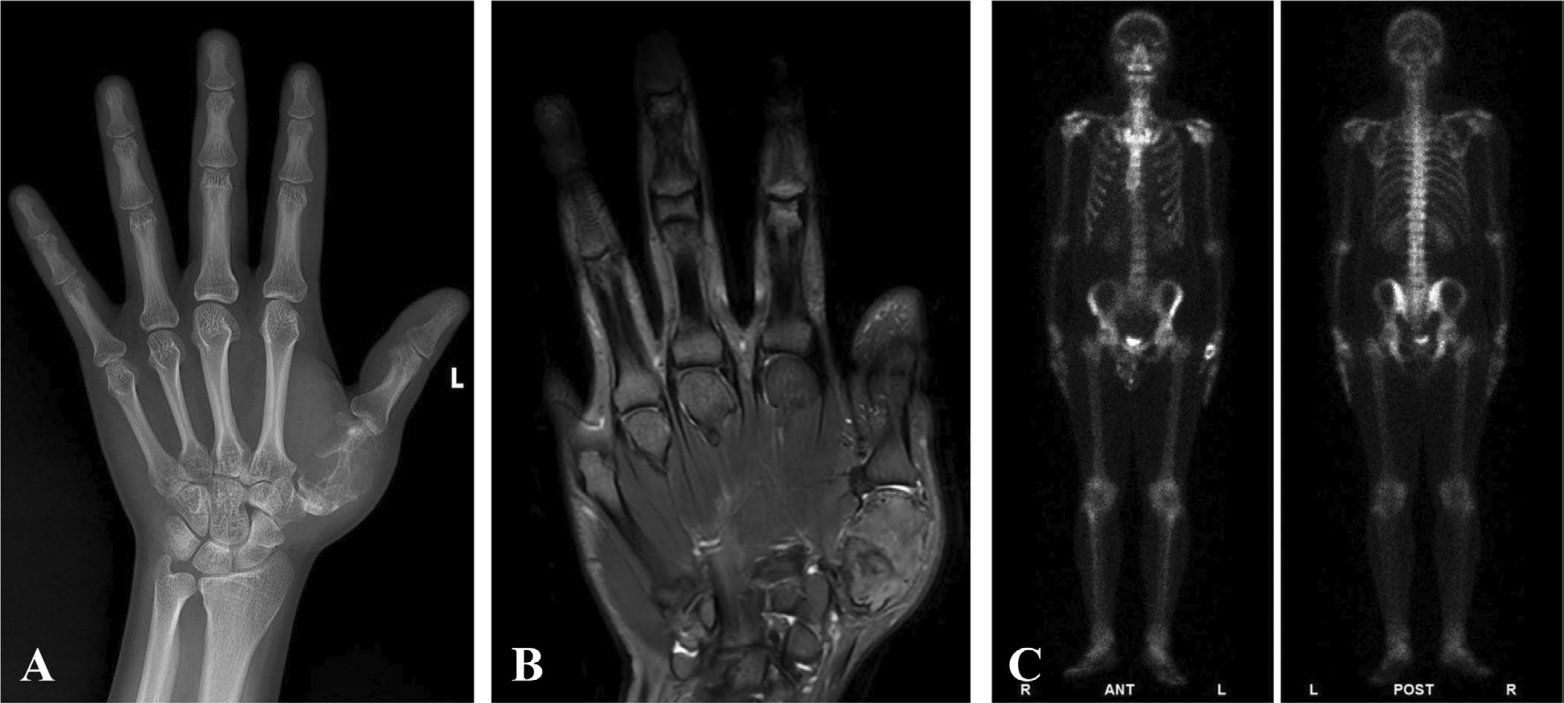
The X‐ray (A) and the MRI (B) demonstrated that the tumor involved the first metacarpal. The SPECT (C) showed no distal metastasis.
